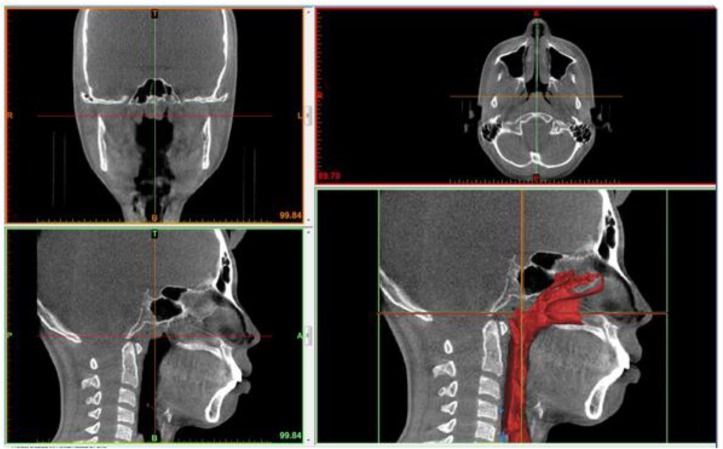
Clinical radiograph of case using Myobrace (T4A^TM^): after treatment CBCT
